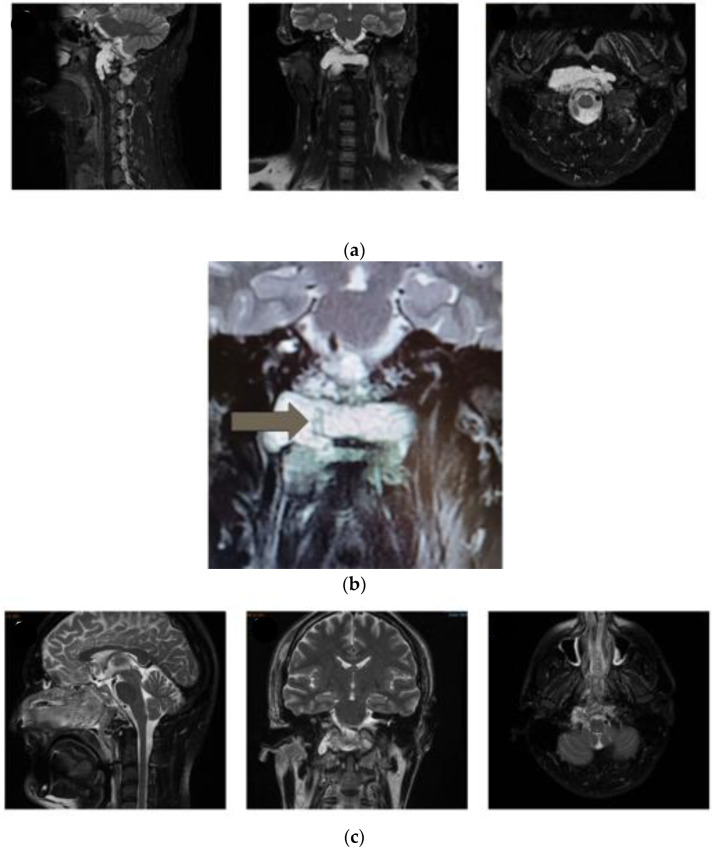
(a) Preoperative images of patient 3, showing the lesion involving the inferior clivus, right occipital condyle, and atlas (arch and right articular mass). (b) MRI obtained after the first unsuccessful attempt at resection via a submandibular approach, showing the biopsy tract (arrow). (c) Postoperative MRI after transoral–transnasal resection, showing near-total resection of the tumor with a small residue in the right lateral mass of the atlas (right image).
